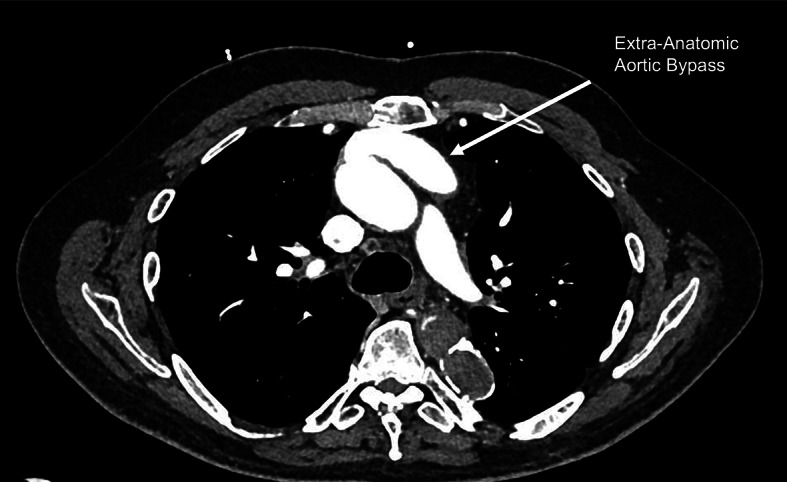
Axial computed tomography image of extra-anatomic aortic bypass sitting behind the posterior sternum
